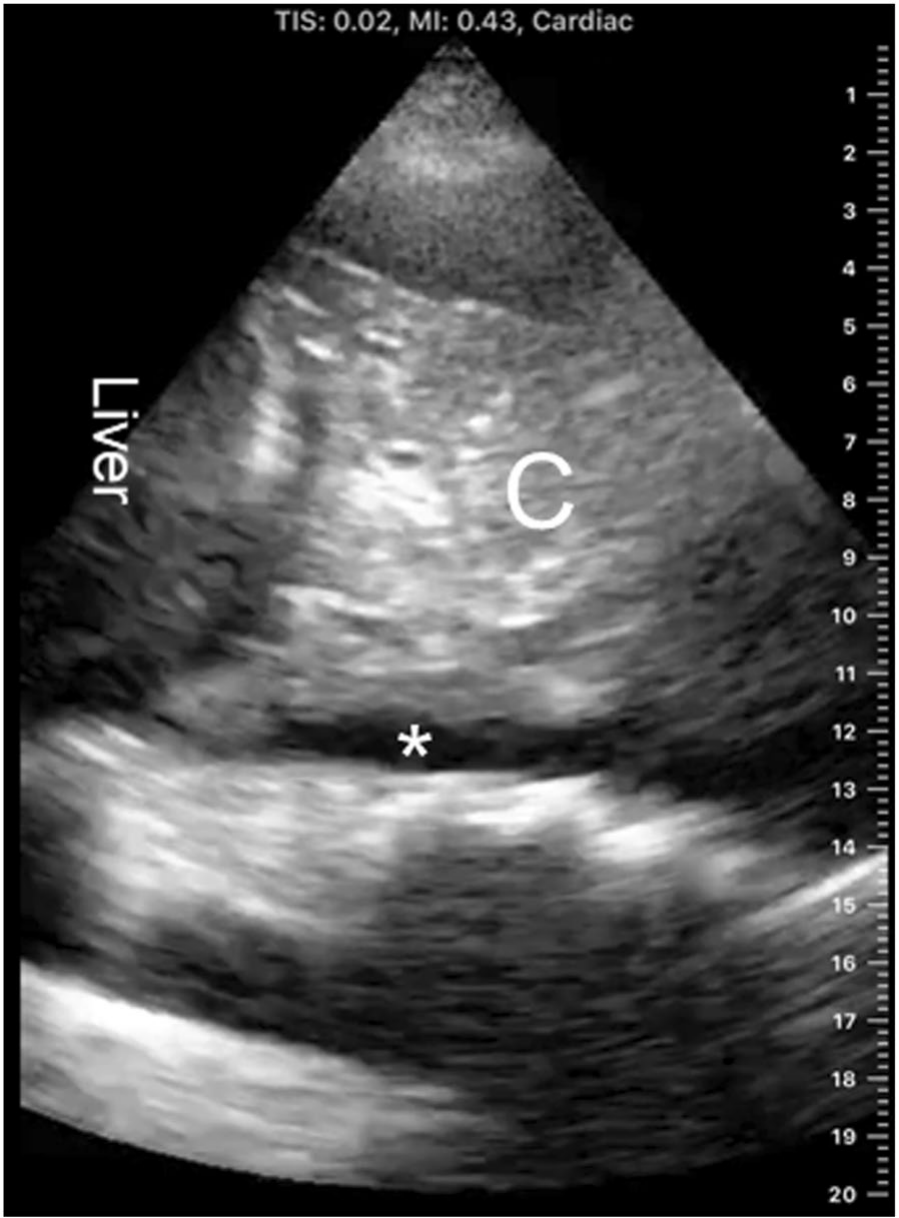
Consolidated lung in a mechanically ventilated patient with intrapulmonary shunt. Consolidated lung in a mechanically ventilated patient with intrapulmonary shunt. Lung ultrasound demonstrating a large area of consolidated lung (C) surrounded by a moderate pleural effusion (*) in zone 6 of the right lung
